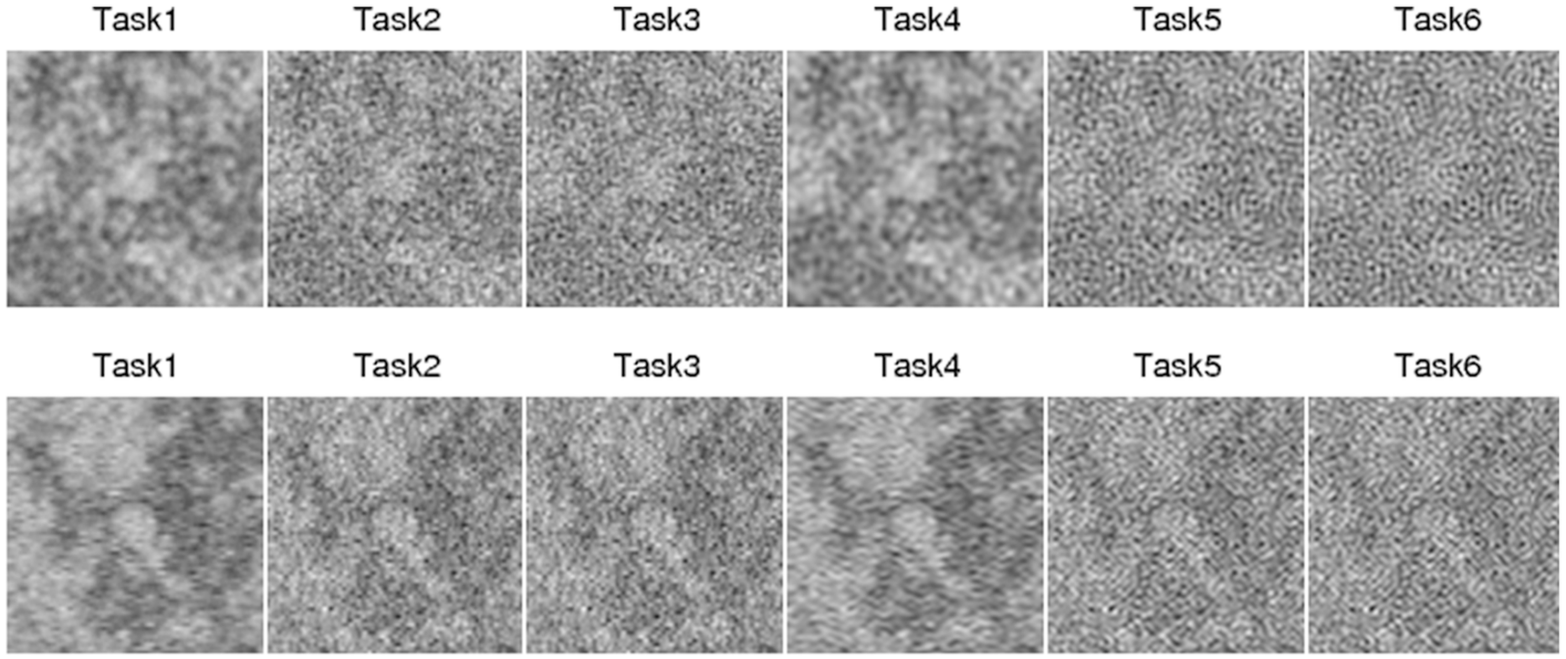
Example CBCT images. Example images with a 2*mm* diameter signal for the transverse (upper) and longitudinal planes (lower).
